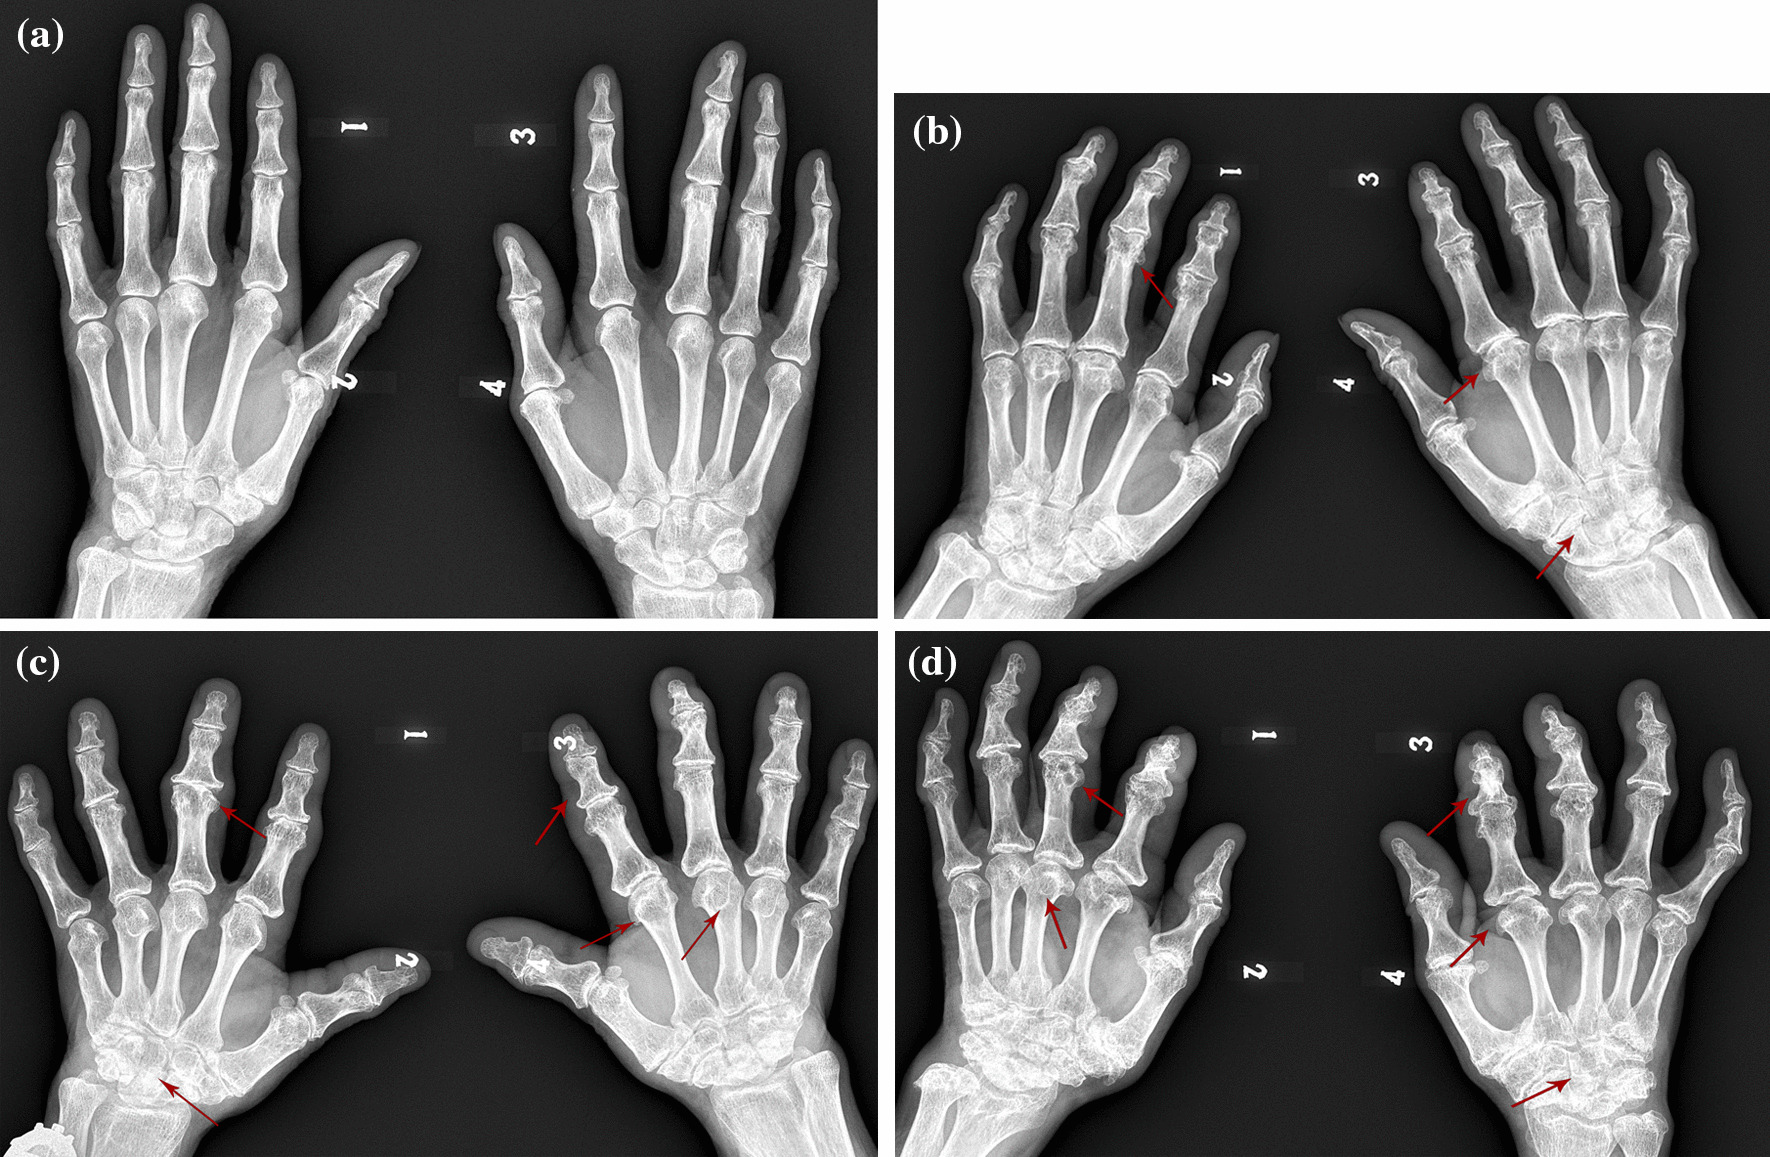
Posterior and anterior X-ray images of both hands in KBD patients and normal controls. a Normal adult hand X-ray image: smooth joint surfaces of palm, finger, wrist, and the joint space is symmetrical and clear. b Hand X-ray images of patients with stage Ӏ: formation of ridge in the middle phalanx, hypertrophy of the tuberosity; pseudocyst of the metacarpal head; crowding and deformation of the carpal bone. c Hand X-ray images of patients with grade II: shortening of the metacarpal phalanx, widening of the base of the phalanx in a triangular shape, and hyperplasia of the edge; swelling of the metacarpal head, pseudocyst, and falciform shadow; crowding and deformation of the carpal bone. d Hand X-ray images of patients with grade III: metacarpophalangeal shortening, widening of the base of the phalanx, bell-shaped changes at the proximal end, hyperplasia of the edge, and articular surface coloboma; metacarpal head swelling, falciform shadow; carpal bone crowding and deformation
